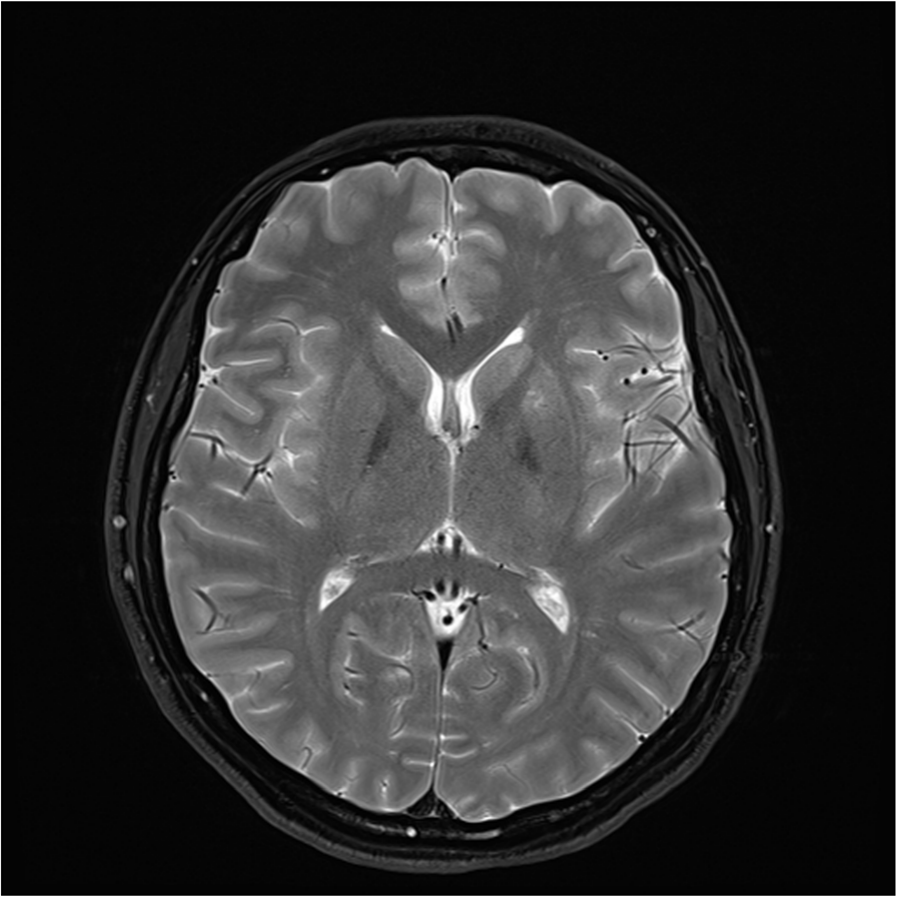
Cerebral MRI axial T2 sequence depicting bilateral engorged pial collateral vessels
